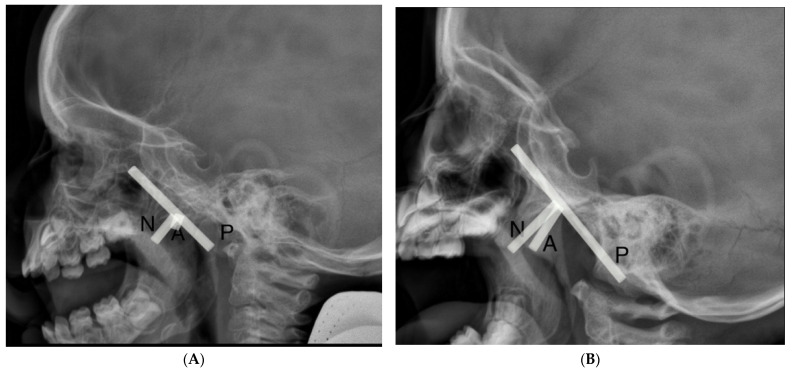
Lateral cephalometric X-ray findings (A) nasopharynx without adenoid hypertrophy, (B) nasopharynx with adenoid hypertrophy. The adenoidal–nasopharyngeal ratio was calculated as the ratio of the distance between the outermost point of the anterior convexity of the adenoid shadow (A) and the straight part of the anterior margin of the basic occiput (P) to the distance between sphenobasioccipital synchondrosis and the posterior end of the hard palate (N).
